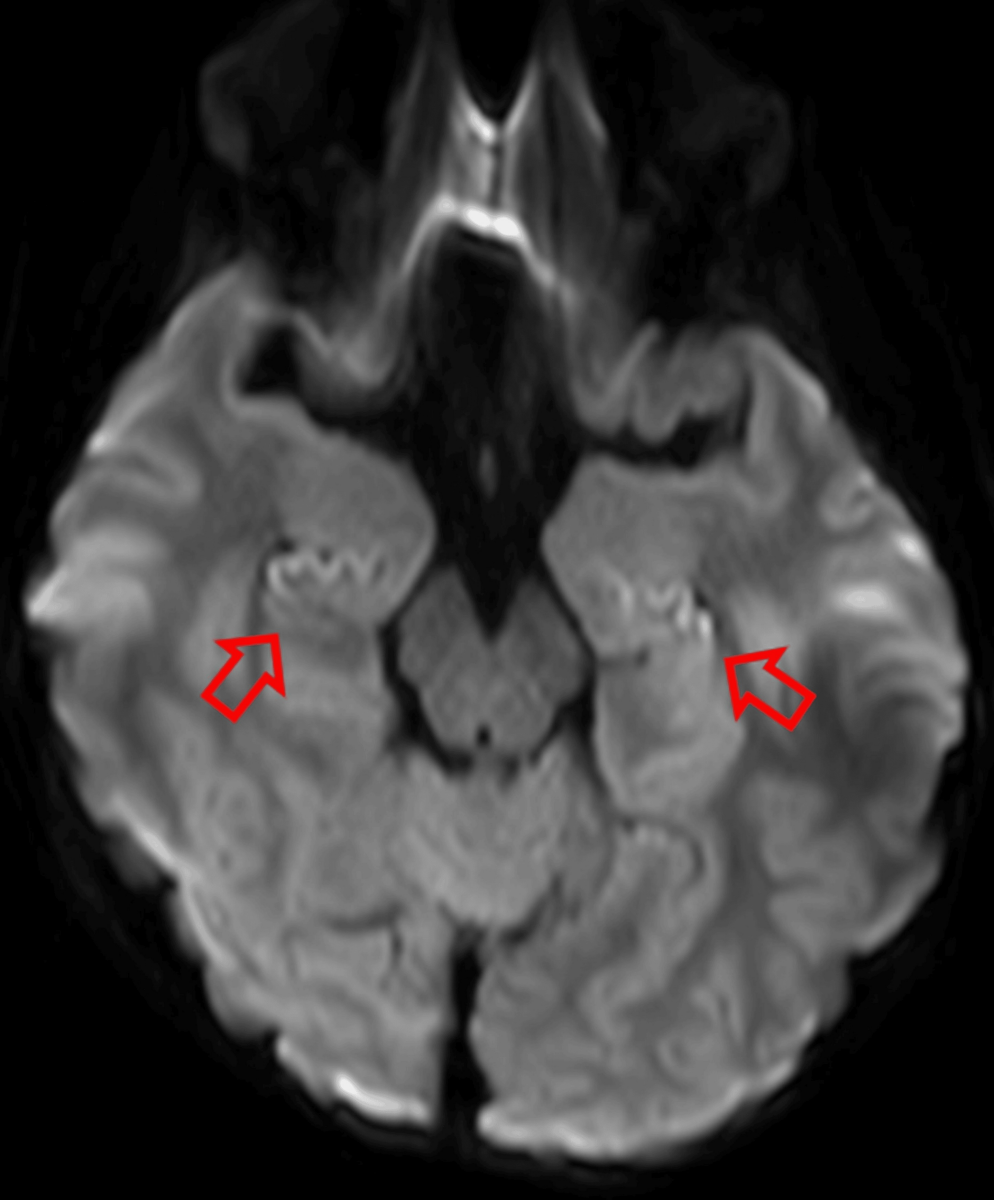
Brain magnetic resonance imaging on day 11 Diffusion-weighted imaging showing hyperintensity in the bilateral hippocampus (red arrows).
